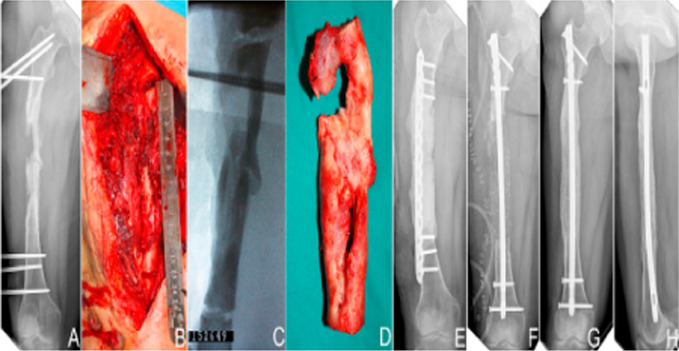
A, Preoperative AP radiograph of posttraumatic femoral infection demonstrating notable bone loss secondary to débridement in a 30-year-old female patient. B and C, Segmental resection of infected bone (aggressive surgical débridement) according to intraoperative fluoroscopy location. D, Excision of infected bone. E, First stage postoperative AP radiograph demonstrated that bone defect was filled with antibiotic bone cement spacer, and locking compression plate was used to limb stabilization after débridement. F, Second stage postoperative AP radiograph demonstrating placement of iliac crest autograft after removal of bone cement and replacement of locking compression plate with intramedullary nail to limb stabilization. G and H, AP and lateral radiographs showing bony consolidation 15 months after bone grafting. The figure reproduced with permission from Han et al.^30^
